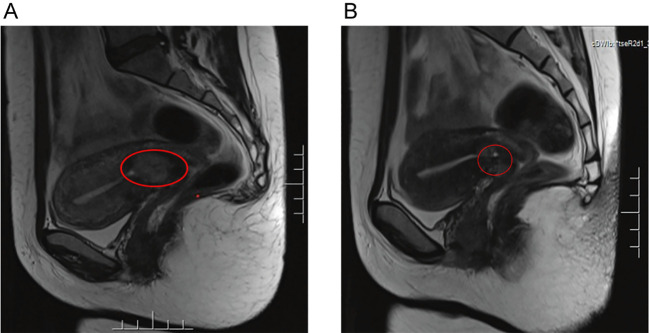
Pelvic MRI shows a lesion centered around the cervix on sagittal T2-weighted Turbo Spin Echo (TSE) image (A). Following chemotherapy, the lesion was significantly reduced in size on sagittal T2- weighted TSE image (B).
